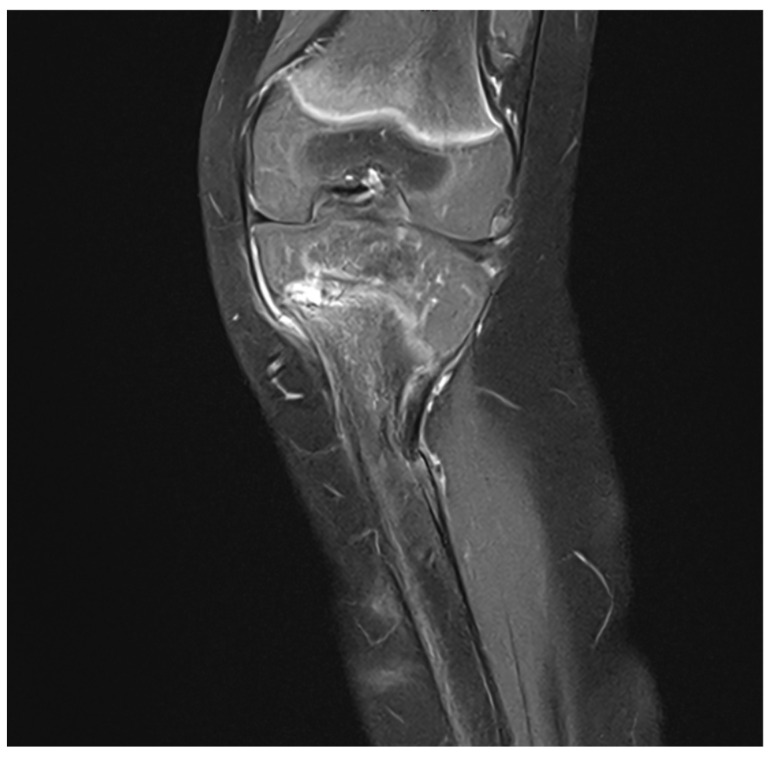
Coronal MRI (T2-weighted fat-suppressed sequence) demonstrating FFCD as a low-signal-intensity lesion in the lateral metaphysis of the left proximal tibia.
